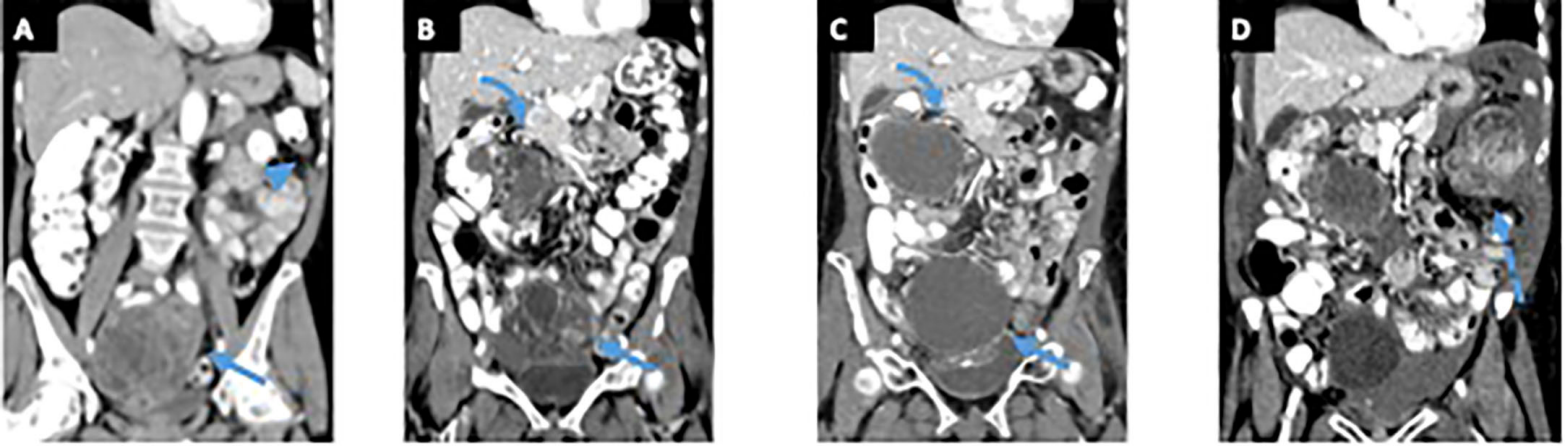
Patient 2. Contrast-enhanced computed tomography (CT) of the abdomen and pelvis at time of the symptoms (A) demonstrates a large pelvic mass with solid and cystic components (arrow), left upper quadrant peritoneal stranding, and peritoneal thickening (arrowhead). Patient underwent exploratory laparotomy revealing a ruptured pelvic mass and a small bowel tumor. After treatment with vincristine/adriamycin/cytoxan/olaratumab, the patient started treatment with pazopanib and underwent palliative radiation to the pelvis. Contrast-enhanced CT of the abdomen and pelvis obtained before patient started treatment with pazopanib (B) shows an enhancing solid and cystic pelvic mass (arrow) and an enhancing solid and cystic right upper quadrant mass (curved arrow), both showing decreased enhancement on contrast-enhanced CT obtained 17 months after starting treatment (C). A contrast-enhanced CT of the abdomen and pelvis obtained 23 months after starting pazopanib (D) and shows a new large enhancing solid and cystic left upper quadrant mass (dashed arrow) suggesting progression of disease. .
